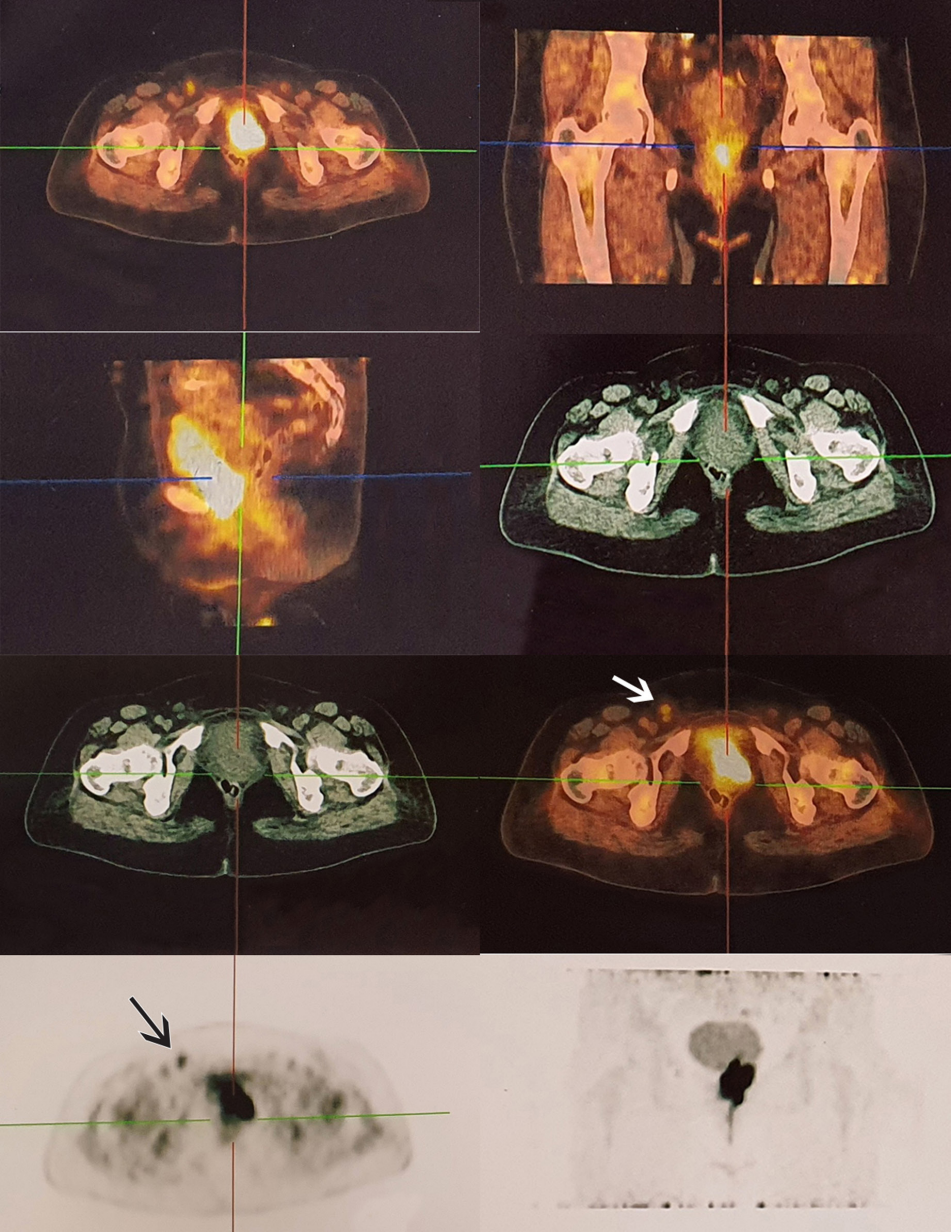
FDG‐PET/CT scan shows a hypermetabolic tumoral mass in the urethra with invasion to the vagina. Few hypermetabolic lymph nodes are noted in bilateral inguinal regions, more prominently on the left side in favor of lymph node metastasis.
